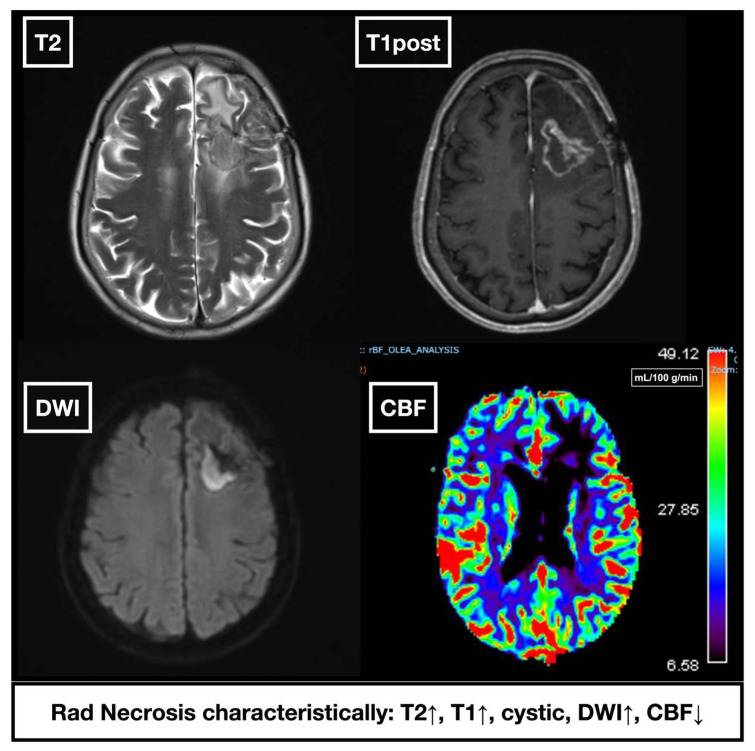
MRI and perfusion imaging features of radiation necrosis. Axial T2, post-contrast T1, and DWI, magnetic resonance sequences, as well as CBF show characteristic findings: T2 and DWI hyperintensity, post-contrast ring enhancement, and decreased cerebral blood flow on perfusion imaging.
